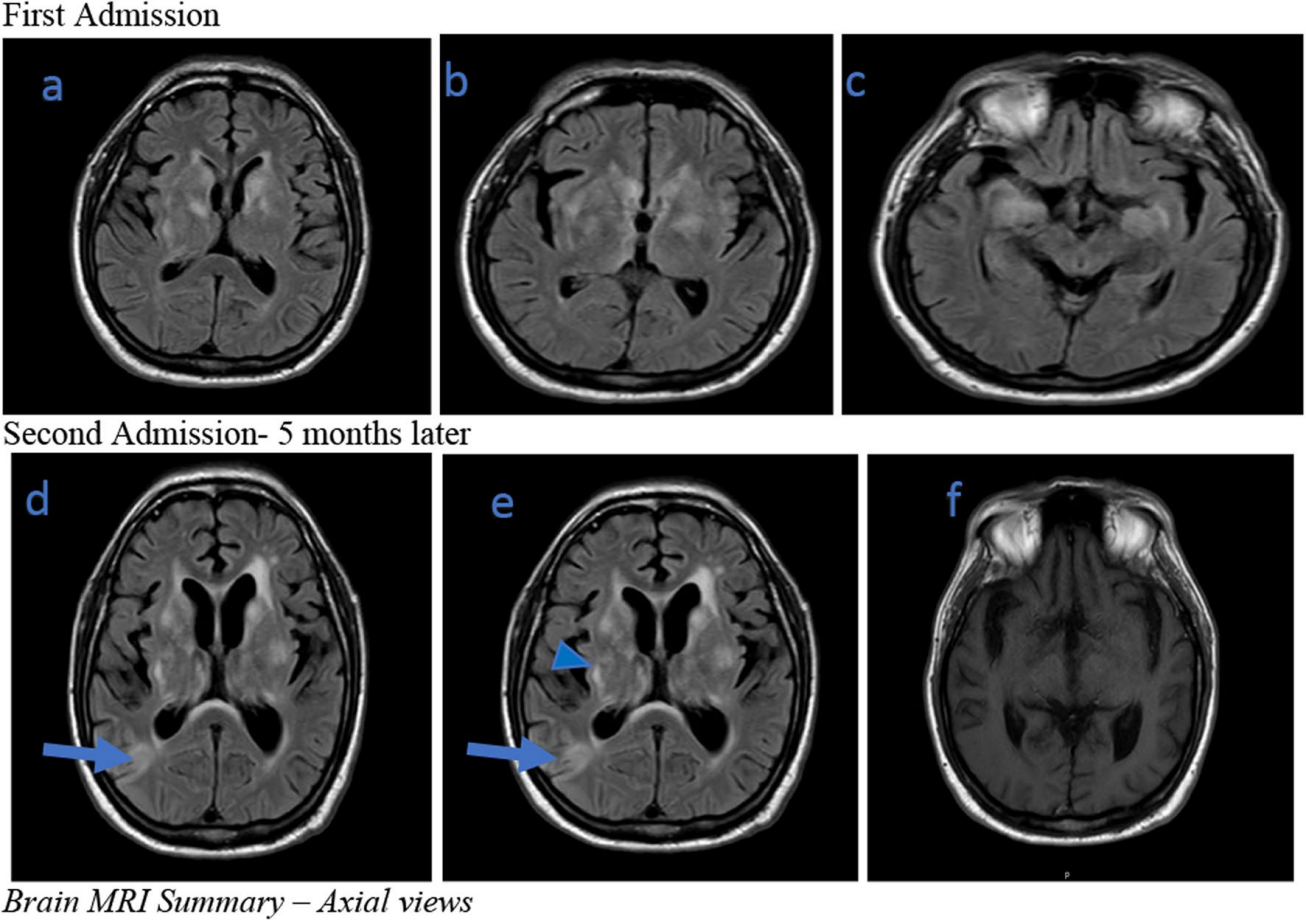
Brain MRI. Brain MRI summary—Axial views, Images a, b, c are images taken on initial presentation. Images d, e, f were taken at his second visit). Arrowhead: Generalized perivascular enhancement in the supratentorial brain during the second admission relative to first admission. There is faint non-specific enhancement in the periventricular regions and pachymeninges. There is progressive patchy prolongation in the white matter as well as deep gray matter structures. Blue arrows point to new infiltrates that were biopsied
